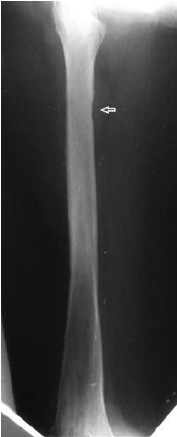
Plain x-rays of the femur
